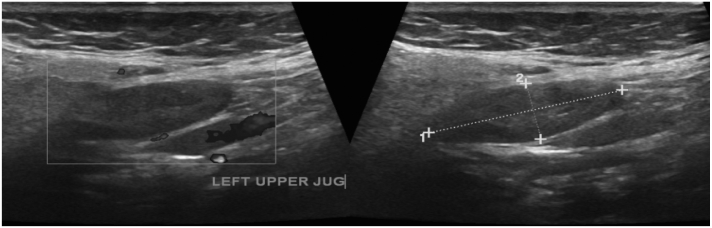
Ultrasound of the neck at upper jugular level showing mildly enlarged hypoechoic left upper jugular lymph node with absence of fatty hilum.
